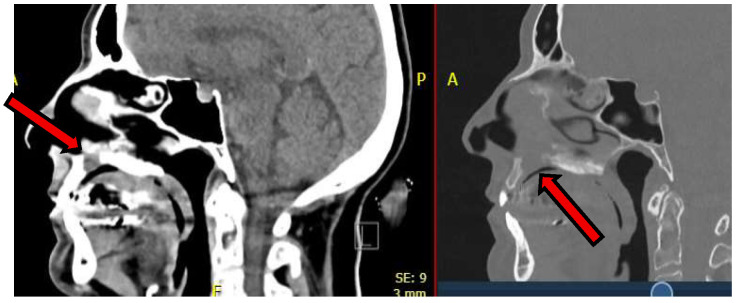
Sagittal computed tomography (CT) imaging demonstrating the anterior hard palate lesion (red arrow).
